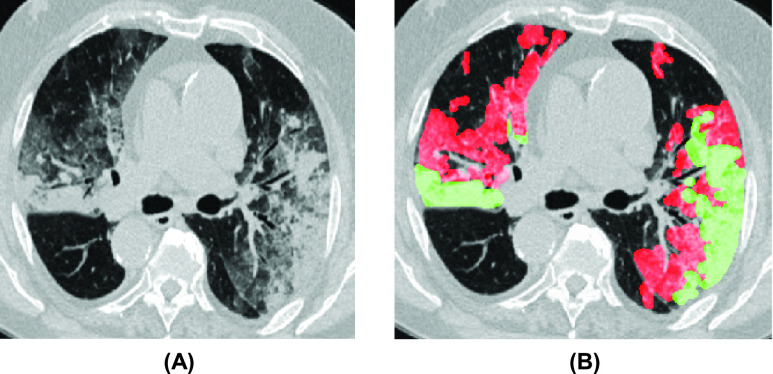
Examples of CT(B) images and original CT images (A) of COVID-19 lesions. The red and green masks represent GGO and solids, respectively. This image is from [11].
